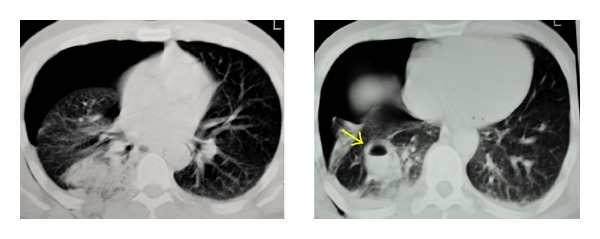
Chest CT scan showing a right-sided pneumothorax and an oval shaped lesion with an air fluid level (foreign body) (yellow arrow).
